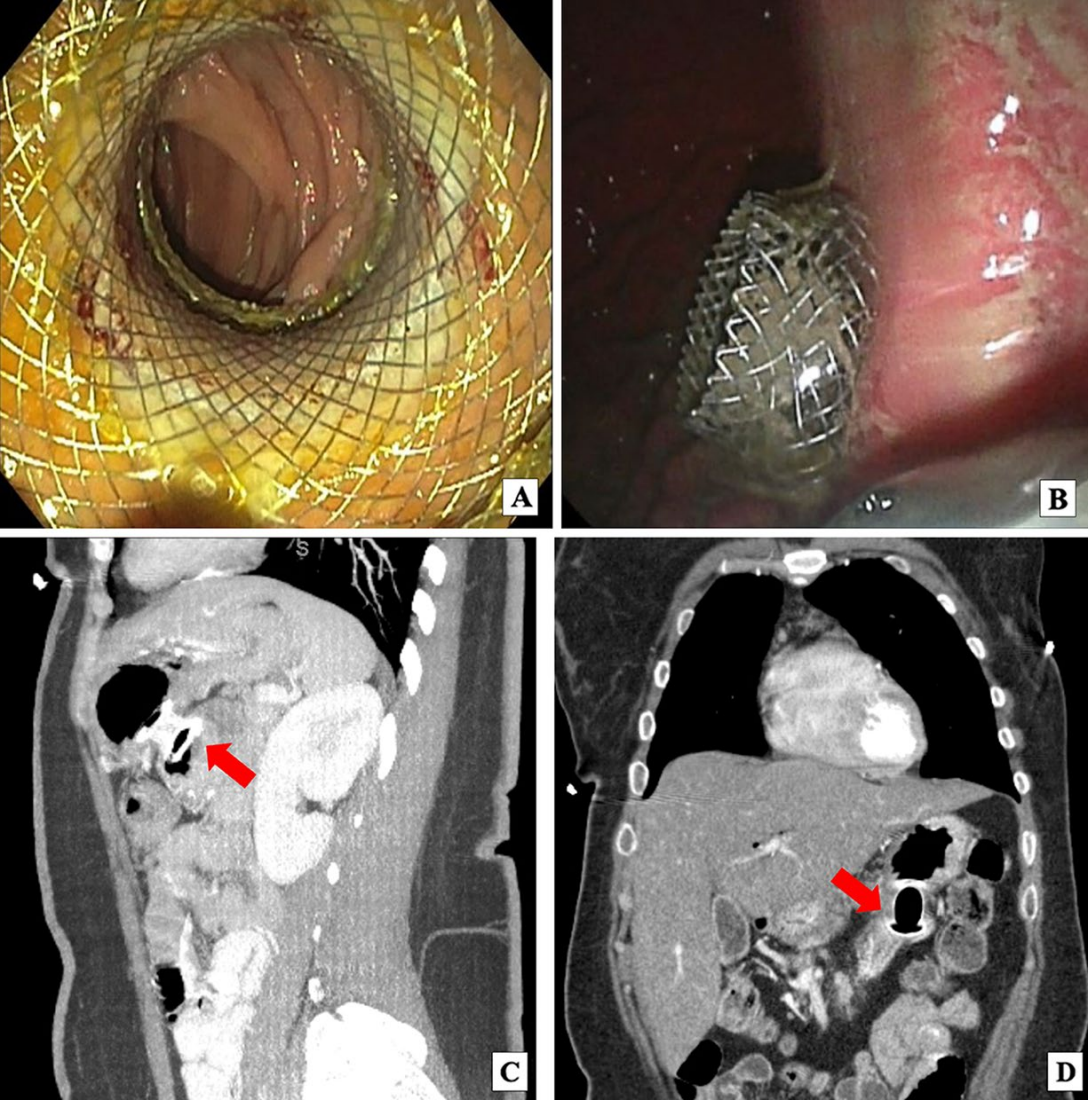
Endoscopic images of lumen apposing metal stent (LAMS) deployment between stomach and jejunum and abdominal CT images with intravenous contrast demonstrating LAMS gastrojejunostomy (GJ). A Jejunum as seen through the LAMS. After contrast was injected to identify the jejunal lumen distal to the duodenal stent that had become stenosed, a 20 mm × 10 mm electrocautery-enhanced LAMS (Hot Axios, BSCI) was deployed from the stomach to the small bowel just distal to the duodenal stent. The LAMS was dilated to 18 mm using a wire-guided balloon dilator. Small bowel was examined distal to the LAMS which was healthy. No trauma from the LAMS was demonstrated. B Deployment of LAMS flange in the stomach. The distance between the gastric and jejunal wall was less than 1 cm. There were no significant blood vessels in the path chosen for LAMS entry. C Sagittal abdominal CT with red arrow pointing to interval LAMS placed between posterior gastric wall and proximal jejunum, distal to the end of the obstructed duodenal stent. D Coronal abdominal CT with red arrow pointing to LAMS between stomach and proximal jejunum
